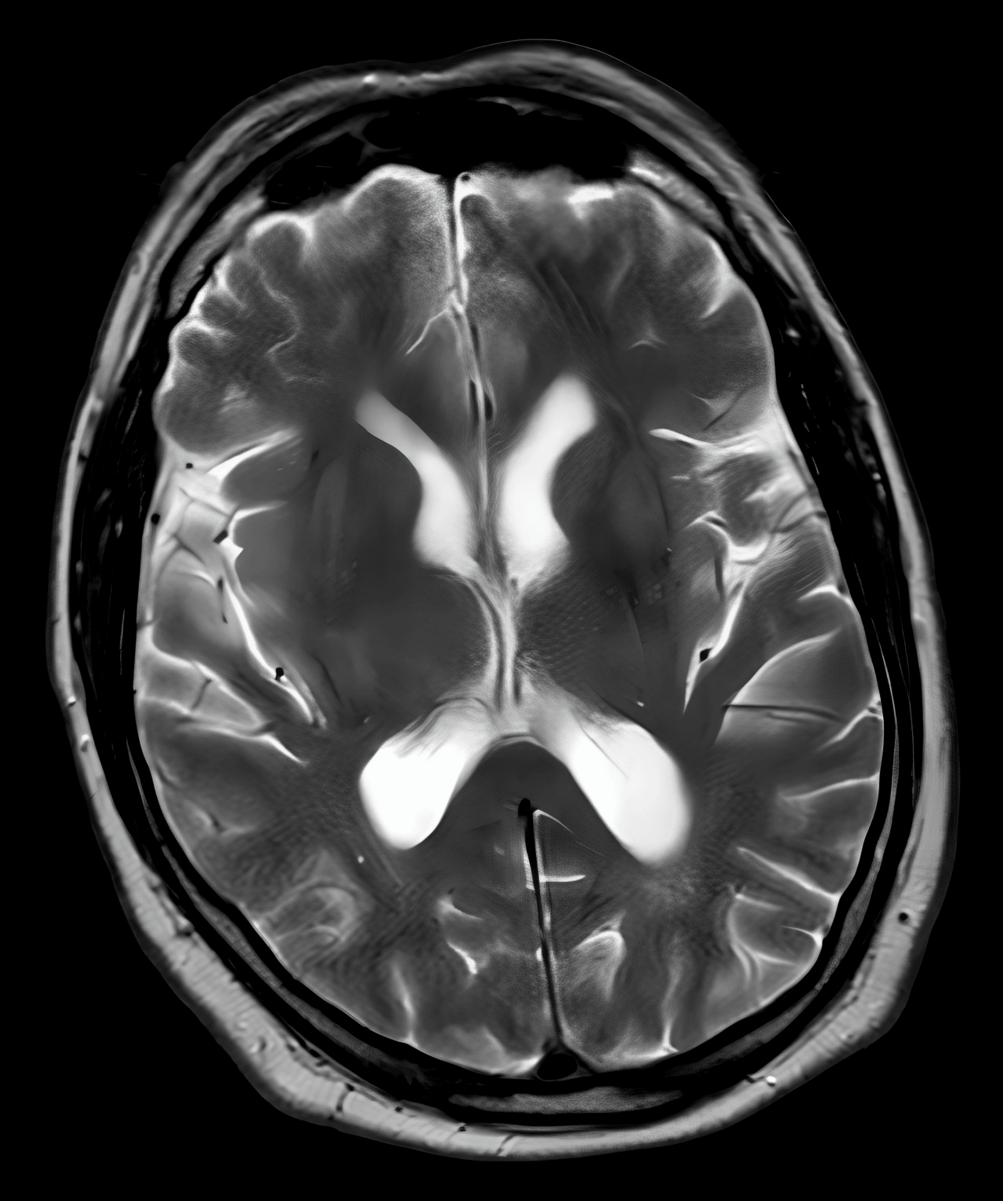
MRI of the head using a T2-weighted fluid-attenuated inversion recovery sequence, demonstrating normal brain parenchyma with no abnormal signal changes.
